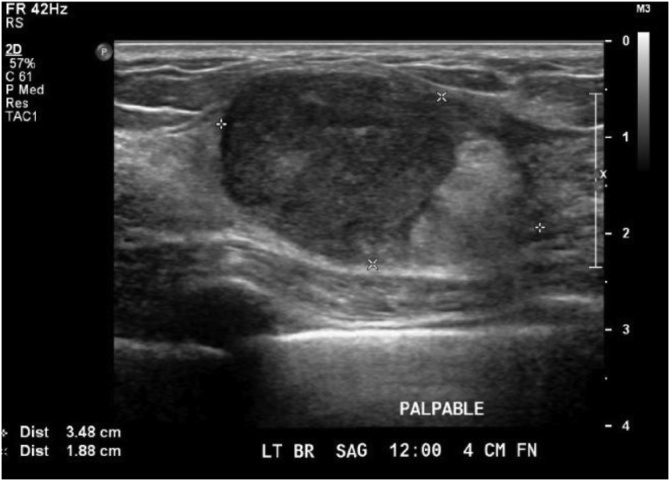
Ultrasound of left breast showing an avascular heterogeneous mass.
